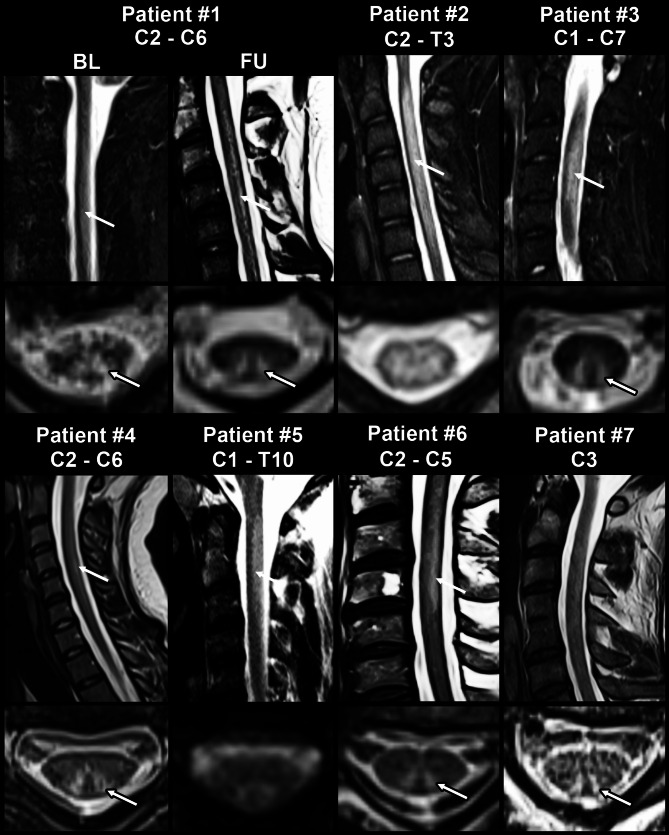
Spinal MRIs of patient #1 – #7 revealed posterior column hyperintensity (white arrow) of the cervical and thoracic spinal cord in T2-weighted midsaggital and T2-weighted axial sections. The extension of the lesion is indicated for each patient, the maximum extension was from C1 to T10. Patient #1, #3, #4, #6 and #7 showed the characteristic “inverted V-sign” on axial sections (white arrow with black contours). Persisting hyperintensities on T2-weighted imaging in patient #1 on follow-up MRI assessment after 4 months. BL: baseline, FU: follow-up
